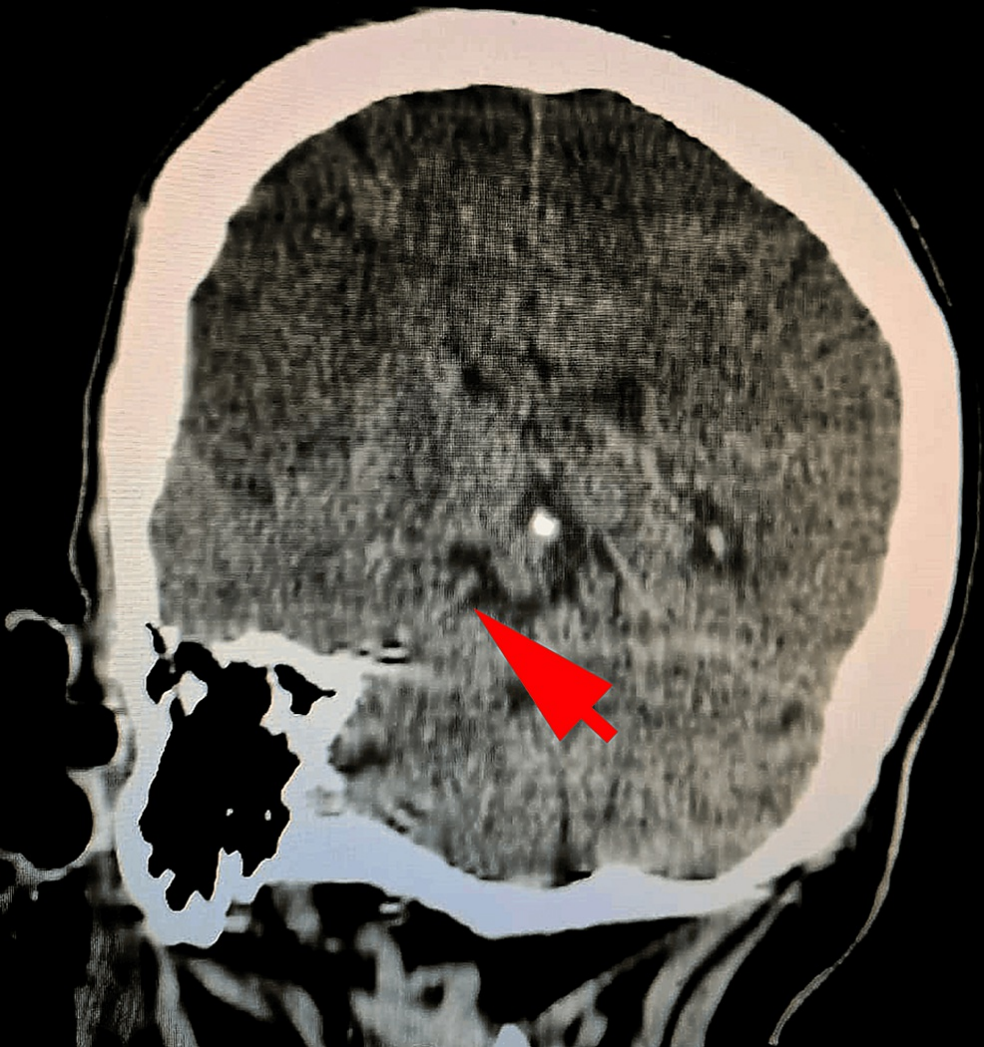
The computed tomography (CT) scan revealed the presence of subfalcine herniation (arrow)
